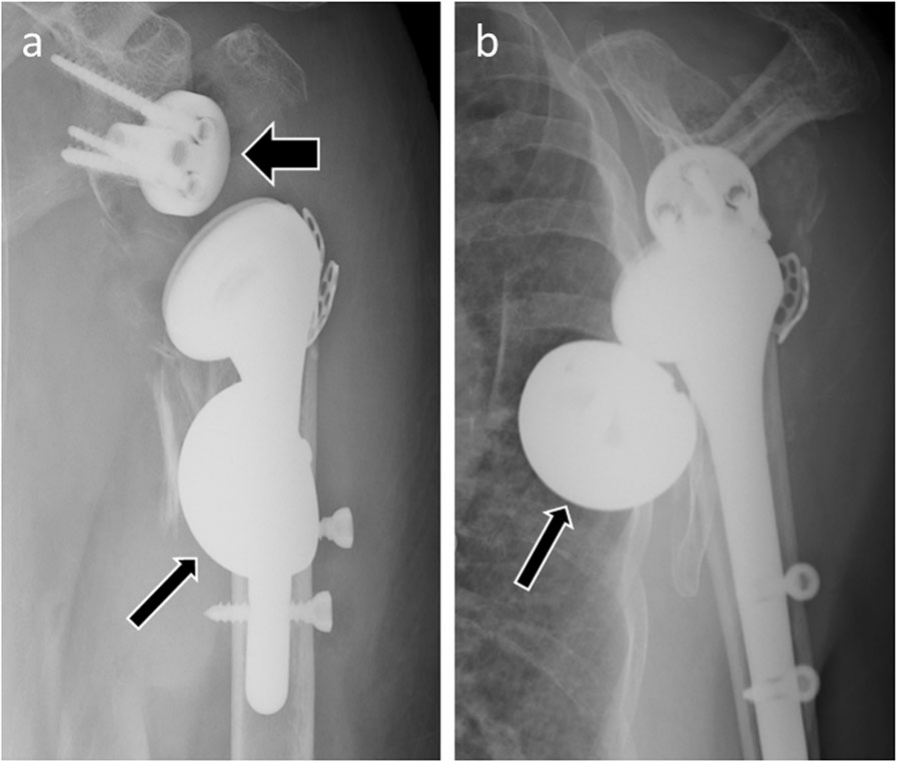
Anteroposterior (a) and scapular Y (b) radiographs of a 87-year-old woman, with reverse shoulder arthroplasty placed 4 days earlier, show glenosphere anteroinferior dislocation (thin arrows). Note the emptiness of the metaglene (thick arrow)
